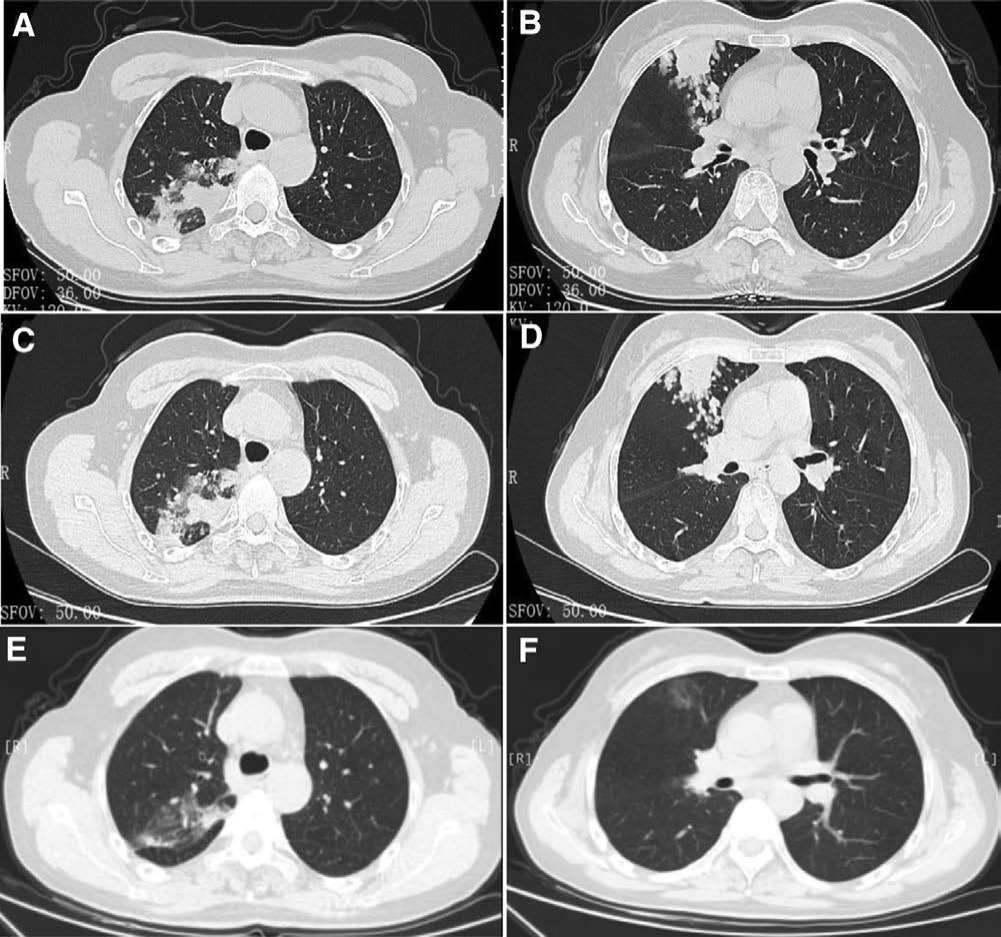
The chest CT scans of the patient. (A and B) The chest CT scan at initial diagnosis. (C and D) The chest CT scan after antibiotic therapy. (E and F) The chest CT scan after 20 days of targeted antitumor therapy. CT = computerized tomography.
